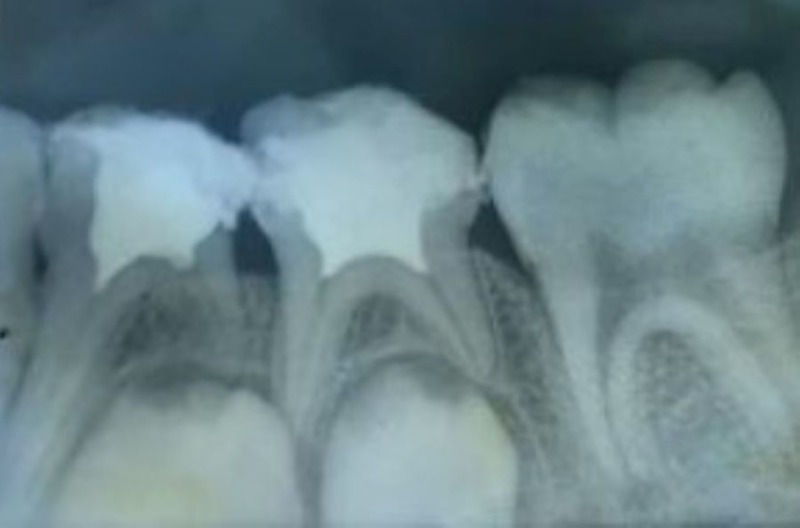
Postoperative radiograph after the placement of MTA MTA - Mineral Trioxide Aggregate
